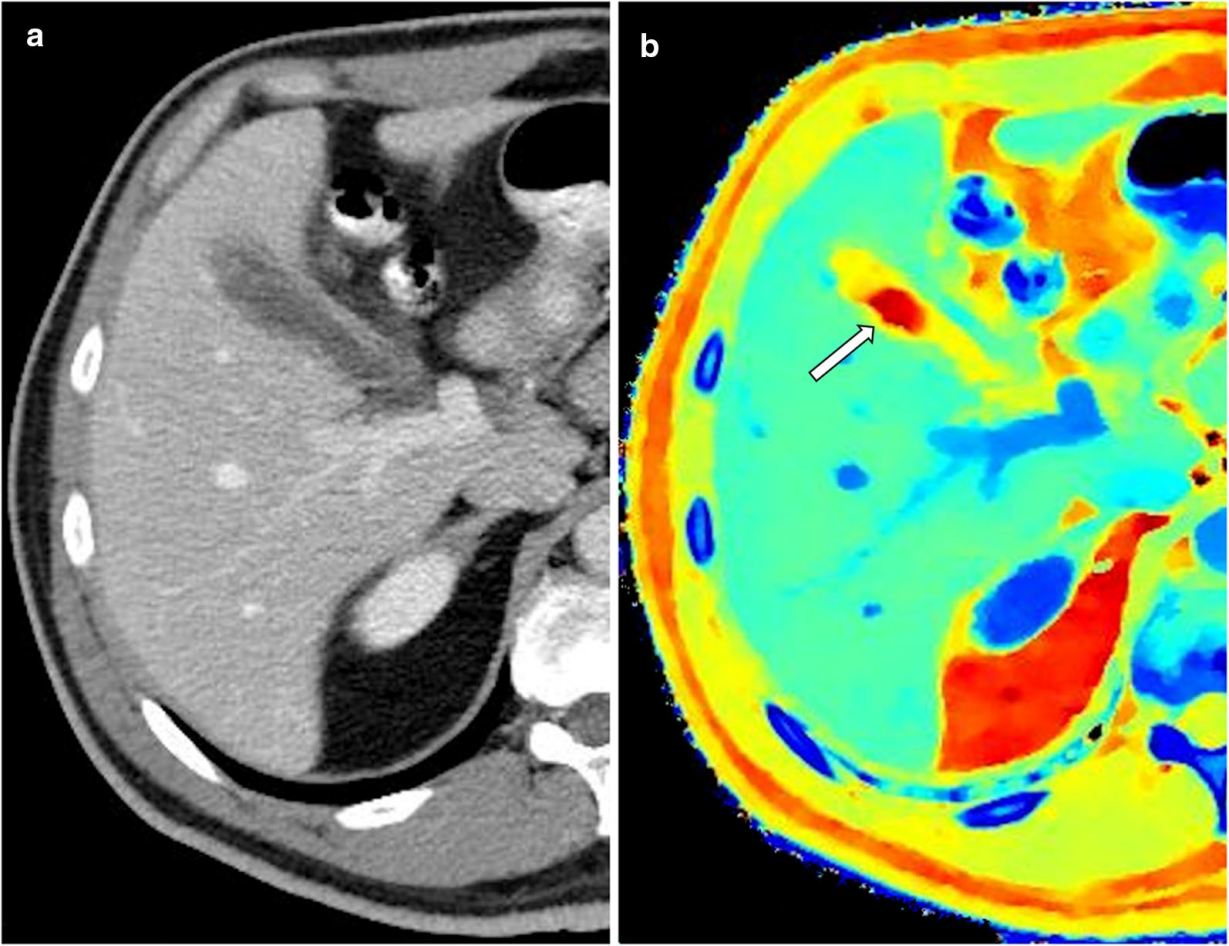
CT of a 64-year-old man showing mild heterogeneity of the gallbladder contents on conventional images (a), with a clear gallbladder stone visible on the effective atomic number map (b) as a focal area with lower atomic number than surrounding bile (arrow)
